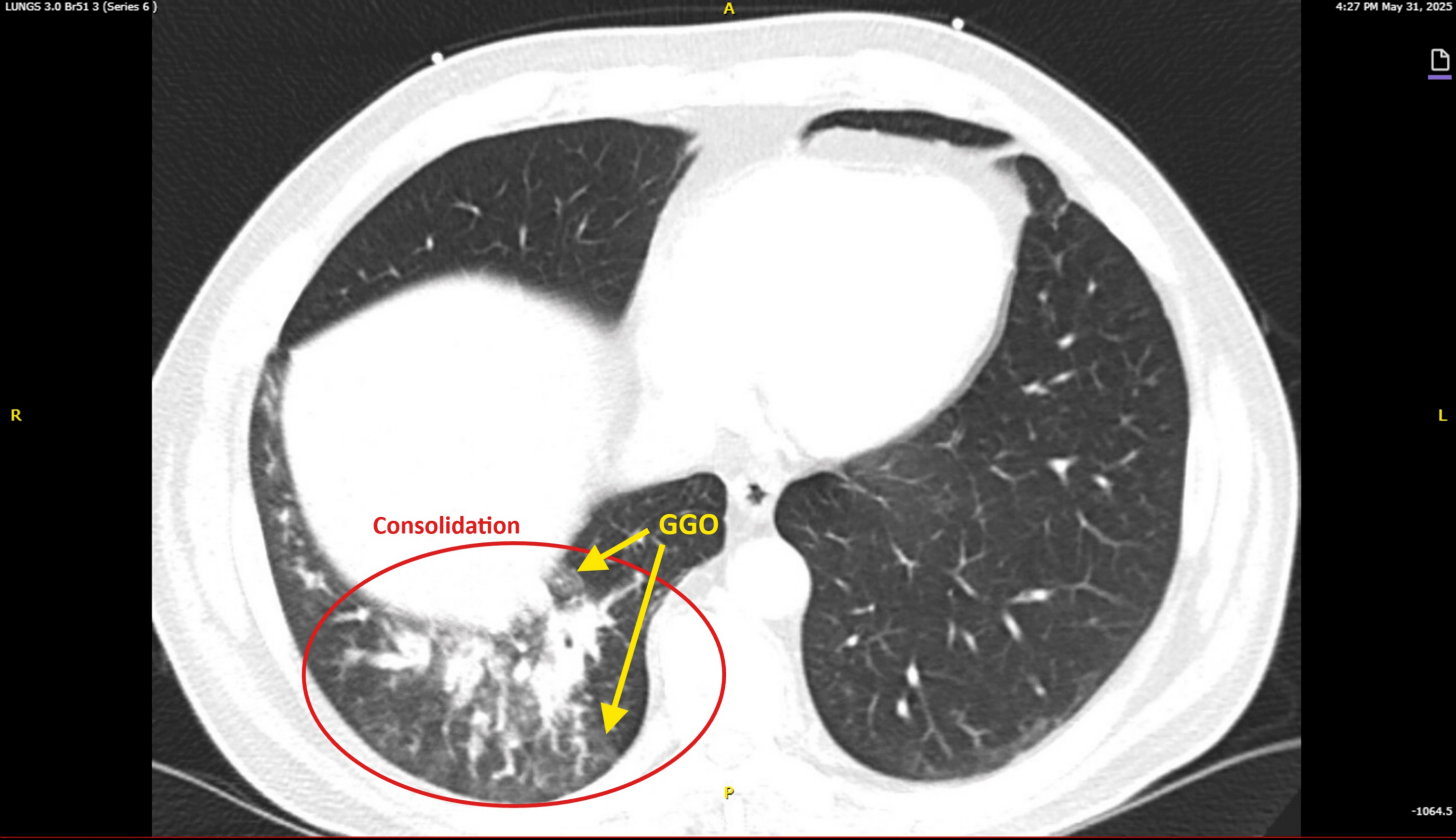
CTA chest, axial view (Series 6, Image 148) taken at a lower thoracic level than Figure 3 demonstrates dense posterior right lower lobe consolidation circled in red, representing the extension of lobar bacterial MSSA pneumonia. Associated GGO highlighted in yellow are consistent with viral influenza A pneumonia. Findings were confirmed by radiological interpretation. CTA: Computed tomography angiography; GGO: Ground glass opacities; MSSA: Methicillin‑sensitive *Staphylococcus aureus*
